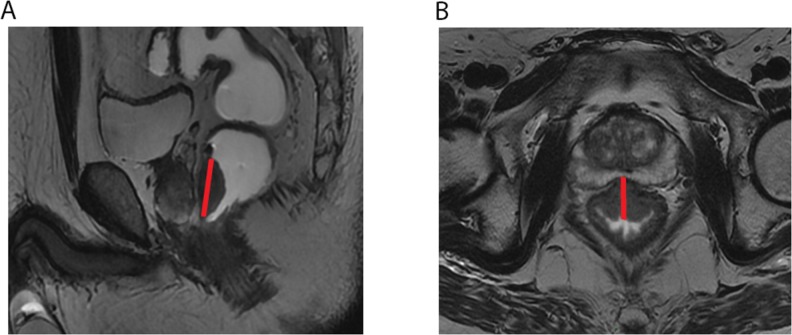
The red straight lines across the lesion indicate the measurement of maximum tumor length (MTL) (A) and maximum tumor thickness (MTT) (B) on sagittal and oblique axial T2-weighted imaging.
